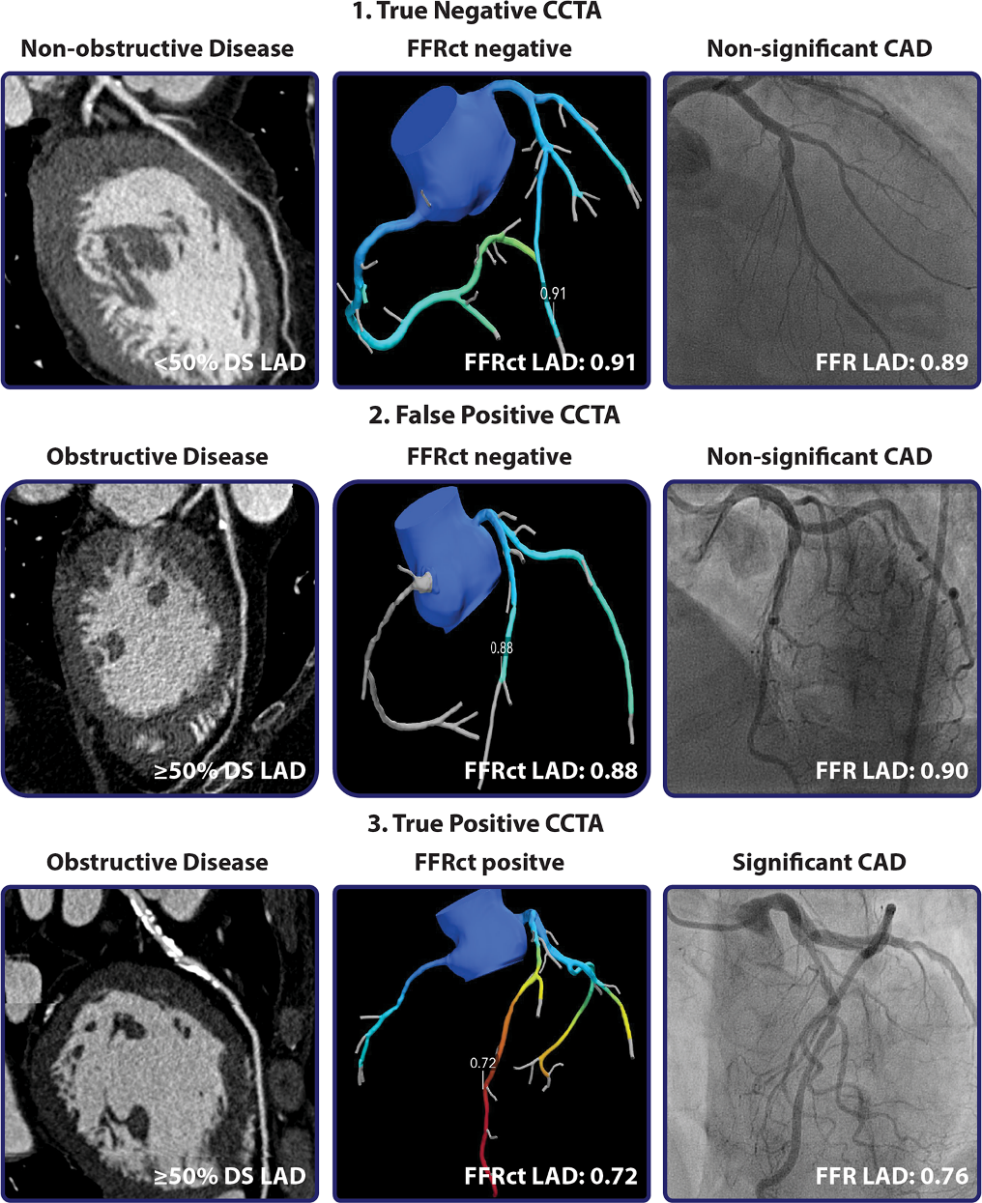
Case examples of CCTA with incorporation of FFRct and the ICA result. Case 1 presents the CCTA of a patient with non-obstructive disease in the LAD, as expected owing to the high sensitivity and negative predictive value of CCTA, subsequent ICA with FFR measurements confirmed non-significant CAD. The diagnostic performance of CCTA is, however, hampered by a relatively high rate of false-positive findings, an example is seen in Case 2. Incorporation of FFRct analysis in the assessment of CCTA can lead to a shift from false-positive results to true negatives (Case 2) and can confirm the significance of CAD as seen in Case 3. *CAD* coronary artery disease, *CCTA* coronary computed tomography angiography, *DS* diameter stenosis, *FFR* fractional flow reserve, *FFRct* CCTA derived FFR, *ICA* invasive coronary angiography, *LAD* left anterior descending artery
